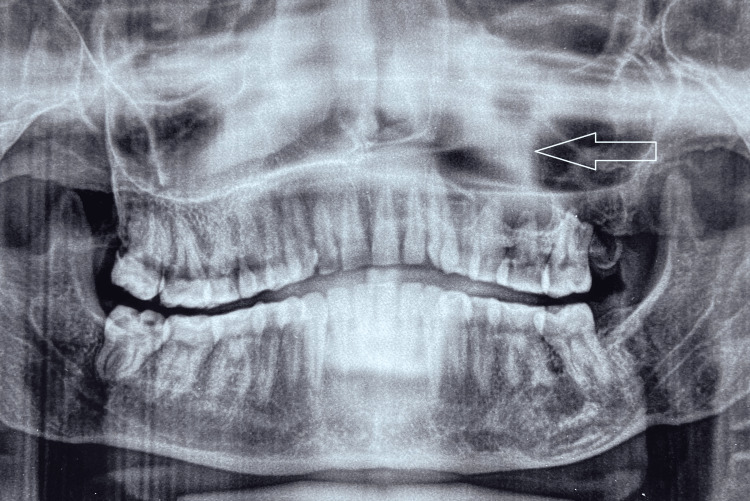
Pre-treatment orthopantomogram showing the expansion of the sinus lining
